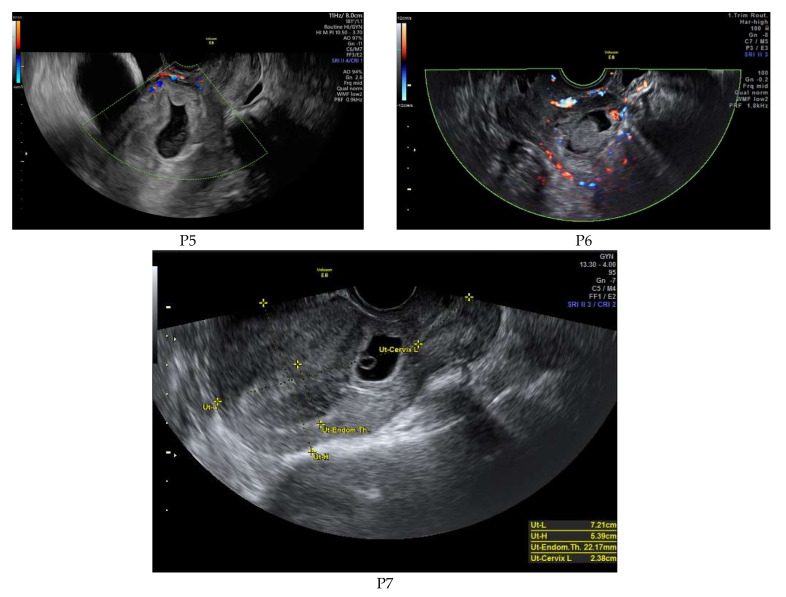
CSP ultrasound imaging for the cases presented in this study before treatment.
